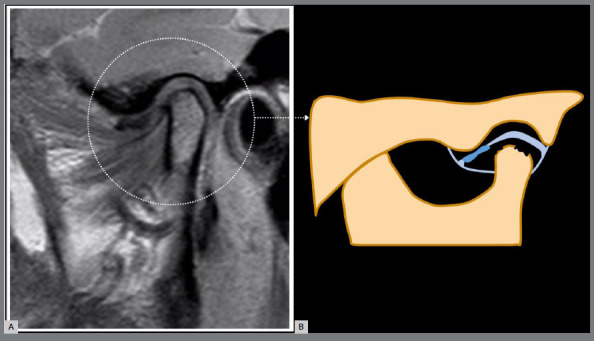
Cartilage erosive phenotype: a loss of continuity of the articular cortex of the condyle can be observed. A) Referential temporomandibular joint MRI sagittal T1-weighted image. B) Temporomandibular joint scheme. Adapted from ROAMES - Rapid OsteoArthritis MRI Eligibility Score (Source: Roemer et al.^8^, 2020).
